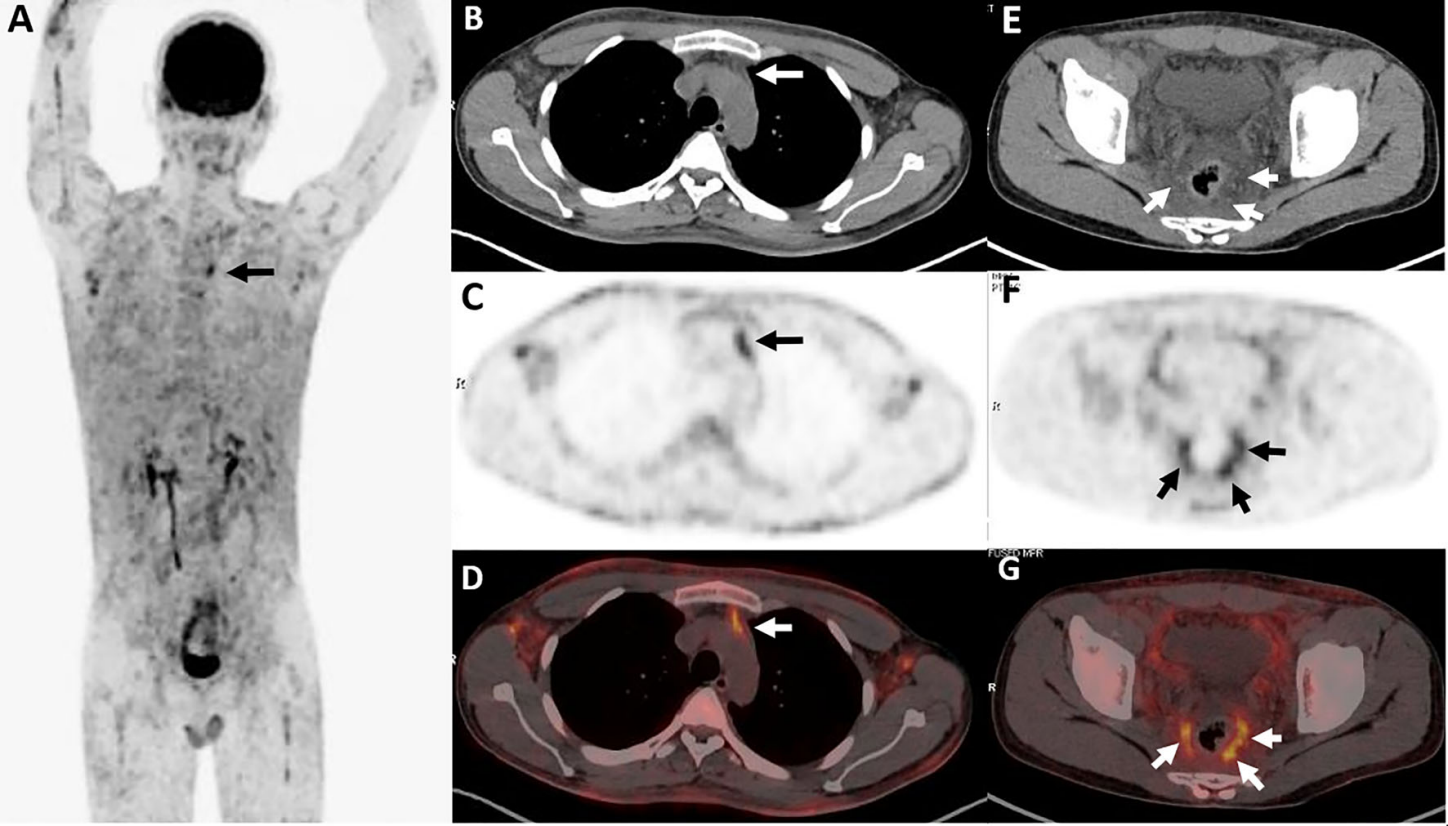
Twenty-five-year-old male with subcutaneous panniculitis-like T-cell lymphoma. Three-dimensional maximum intensity projection PET image (A) shows diffuse hypermetabolic lesions in the subcutaneous of the body. Thoracic axial CT (B), corresponding PET (C) and fusion PET/CT (D) images show a poorly defined lesion with high uptake of ^18^F-FDG in the anterior mediastinum (arrows). Pelvic axial CT (E), corresponding PET (F), and fusion PET/CT (G) images show perirectal lesions are positive on PET scan but negative on CT scan.
